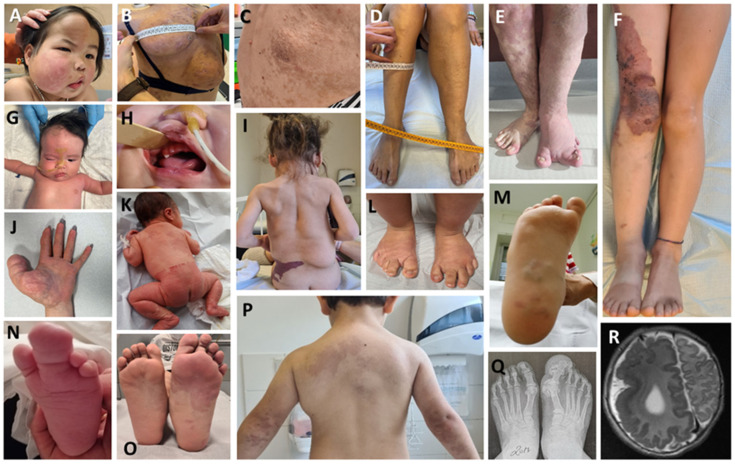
(A) Infiltrating fibro-lipomatous hyperplasia of right cheek and hemiface; (B–D) lipomatous malformation of the back (B) with seborrheic keratosis (C) and muscular hypertrophy of the left hemibody in the same patient (C); (E) Klippel–Trenaunay syndrome (KTS) with fibrose hyperplasia and vascular malformation of the left leg with splayed aspect and syndactyly of the second and third toes of the left foot; vascular malformation with macrodactyly of the fourth toe of the right foot; (F) Klippel–Trenaunay syndrome (KTS); (G,H) infiltrating fibro-lipomatosis of the face (G) with gingival hypertrophy, precocious tooth eruption and bilateral macrodontia (H); (I) congenital lipomatous overgrowth, vascular malformations, epidermal nevi and scoliosis/skeletal/spinal anomalies (CLOVES) with lipomatosis and vascular malformation of the trunk; (J) macrodactyly of the thumb and fibroadipose hyperplasia (FH) of the thumb and palm of the left hand; (K) diffuse capillary malformation with overgrowth (DCMO); (L) fibroadipose hyperplasia of the feet with splayed appearance in a patient with megalencephaly–capillary malformation (MCAP); (M) vascular malformation and FH of the sole of the right foot; (N) macrodactyly and syndactyly of the second and third toe and FH of the sole of the left foot; (O) fibrous hyperplasia with associated vascular malformation of the left foot; (P) CLOVES with lipomatosis and vascular malformation; (Q) X-ray of the feet showing bone and fibrous hyperplasia of the first toe of the left foot; (R) magnetic resonance imaging of the brain showing hemimegalencephaly and lissencephaly of the right hemisphere.
